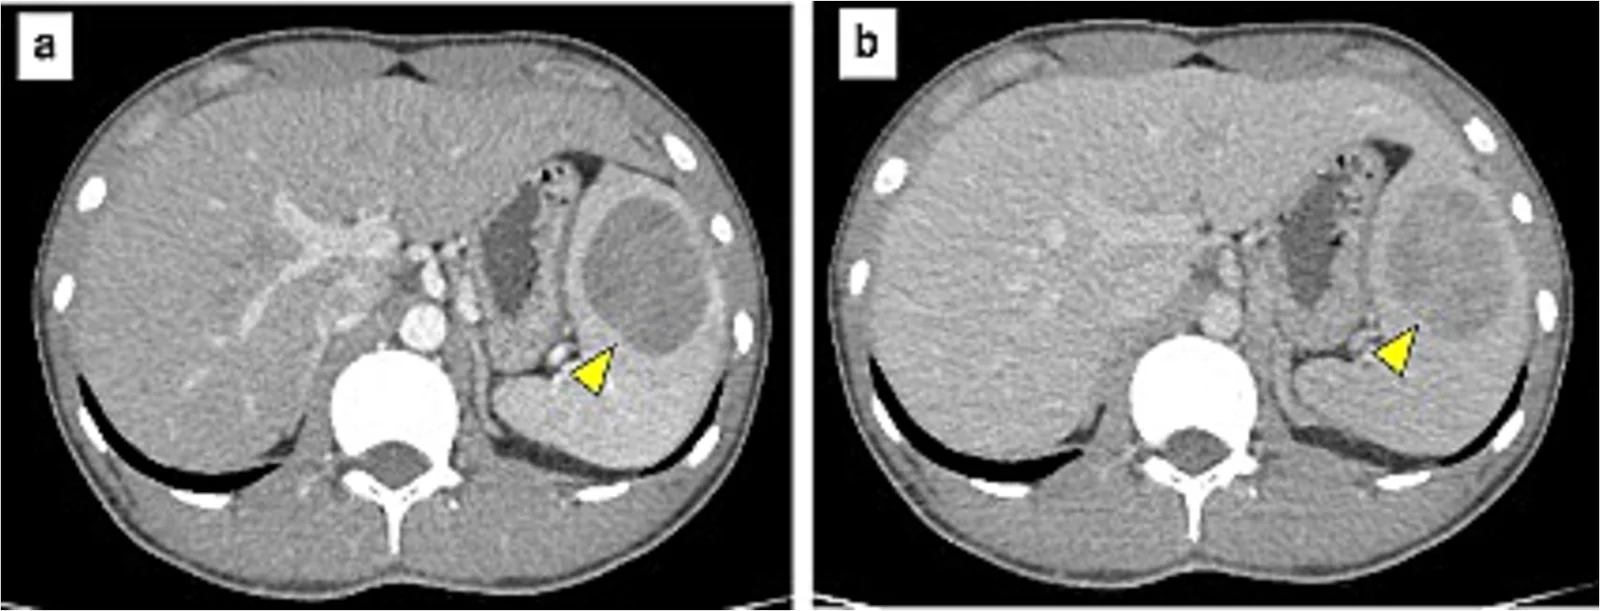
Contrast-enhanced CT. Arterial phase (a) and equilibrium phase (b) images show a well-defined splenic mass with a characteristic spoke-wheel–like enhancement pattern. In the arterial phase, radial linear enhancement is observed from the periphery toward the center of the lesion. In the equilibrium phase, the enhancement progresses centrally.
